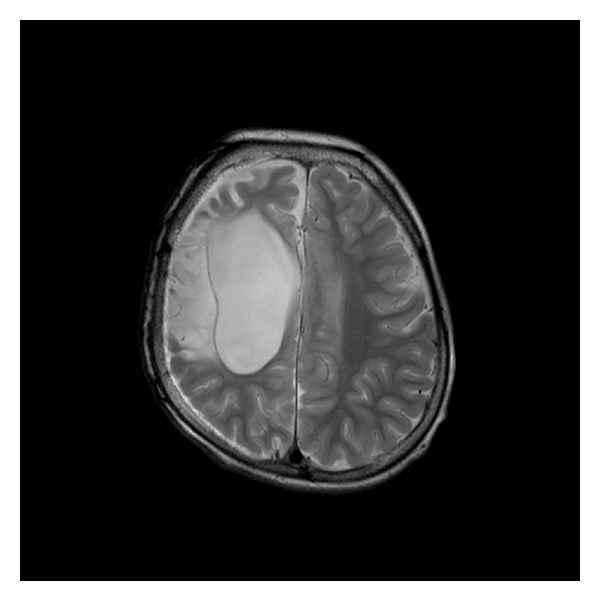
T2W Axial MRI image of a 5-year-old boy who presented with intractable epilepsy secondary to postencephalitic sequelae (PES) causing damage to the right side.
